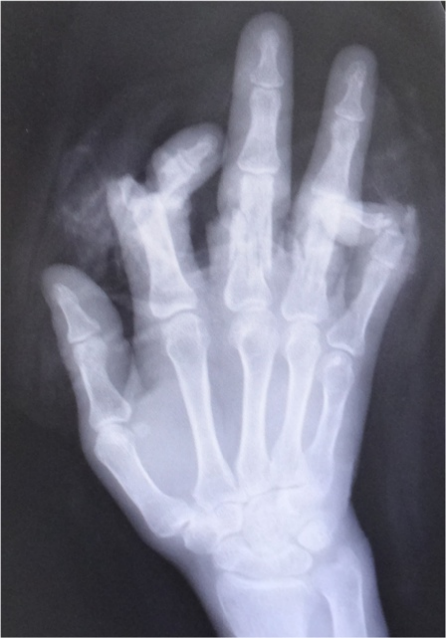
Case 3. An X-ray image of unjured hand
